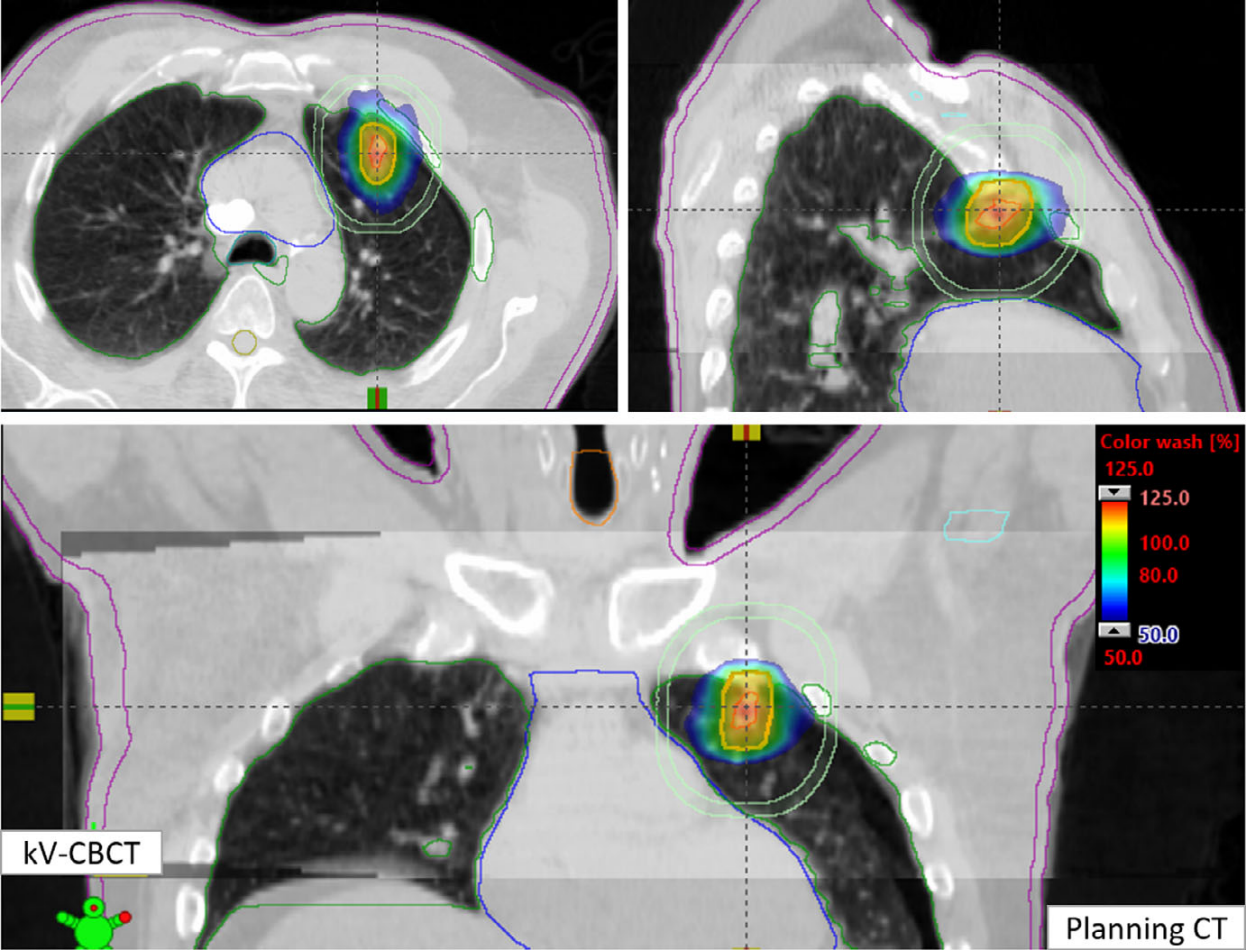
Axial, coronal and sagittal views of Halcyon kV‐CBCT images (see inset) coregistered with planning CT images (see back of coronal and sagittal views) used for image‐guided SBRT on Halcyon. In addition to anatomical landmarks, the planned dose cloud was superimposed for this treatment. Halcyon kV‐CBCT images were acquired in the treatment position in free breathing with abdominal compression. 3D soft‐tissue matching was performed via auto‐registration of online kV‐CBCT with the planning CT followed by manually fine‐tuning for setup corrections.
